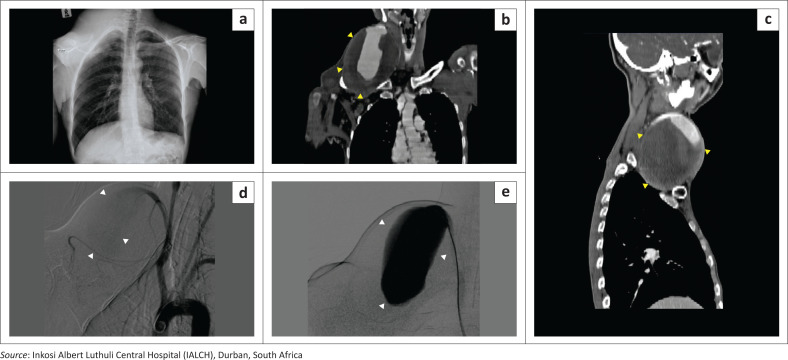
Images from a 41-year-old man with a 10-month history of a rapidly enlarging right-sided neck mass and decreased right upper-limb function. (a) Chest radiograph demonstrating a large soft-tissue mass in the right supraclavicular region without significant tracheal narrowing. (b, c) Coronal and sagittal contrast-enhanced CT angiography revealing a large saccular aneurysm arising from the right subclavian artery, measuring 10 cm, with intraluminal thrombus. (d, e) Digital subtraction angiography demonstrates opacification of the aneurysmal sac (white arrowheads). The patient declined surgical intervention.
